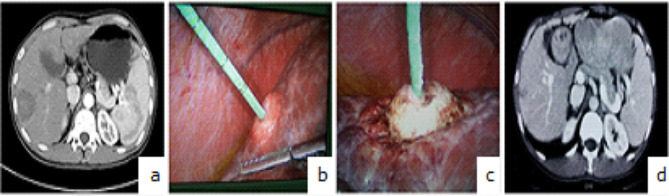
One case treated by laparoscopic-assisted microwave ablation. a: preoperative CT scan, b: antenna inserted into the center of the tumor under laparoscopy, c: the tumor coagulated by microwave, d: CT scan showed the tumor inactivated by microwave
